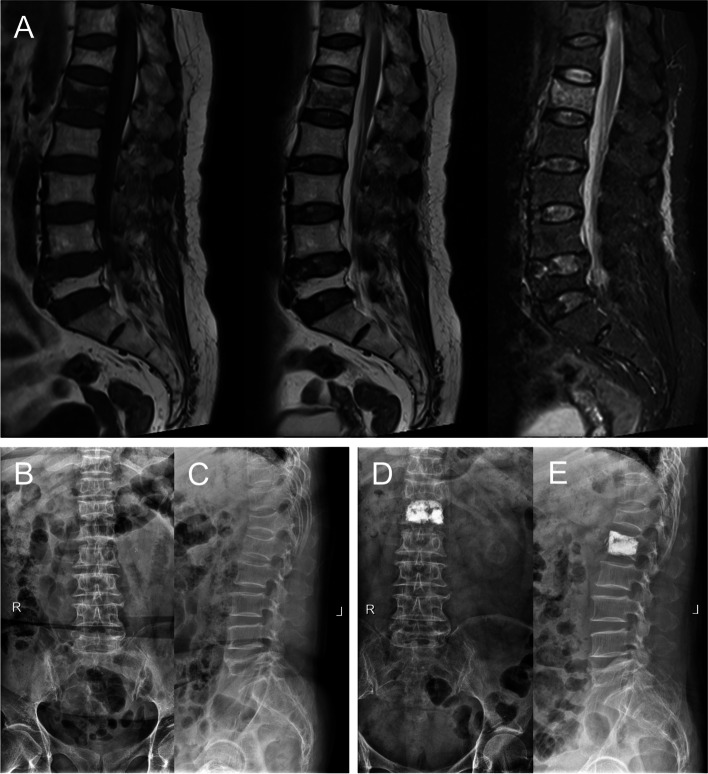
A 59-year-old female patient with back pain for 10 days. The T1-weighted, T2-weighted and T2-weighted fat suppression MR images (A) show L1 vertebral fracture with severe FI. Preoperative anteroposterior (B) and lateral (C) X-ray images show L1 and T12 vertebral compression. Postoperative anteroposterior (D) and lateral (E) X-ray images present satisfactory cement distribution after percutaneous kyphoplasty surgery
